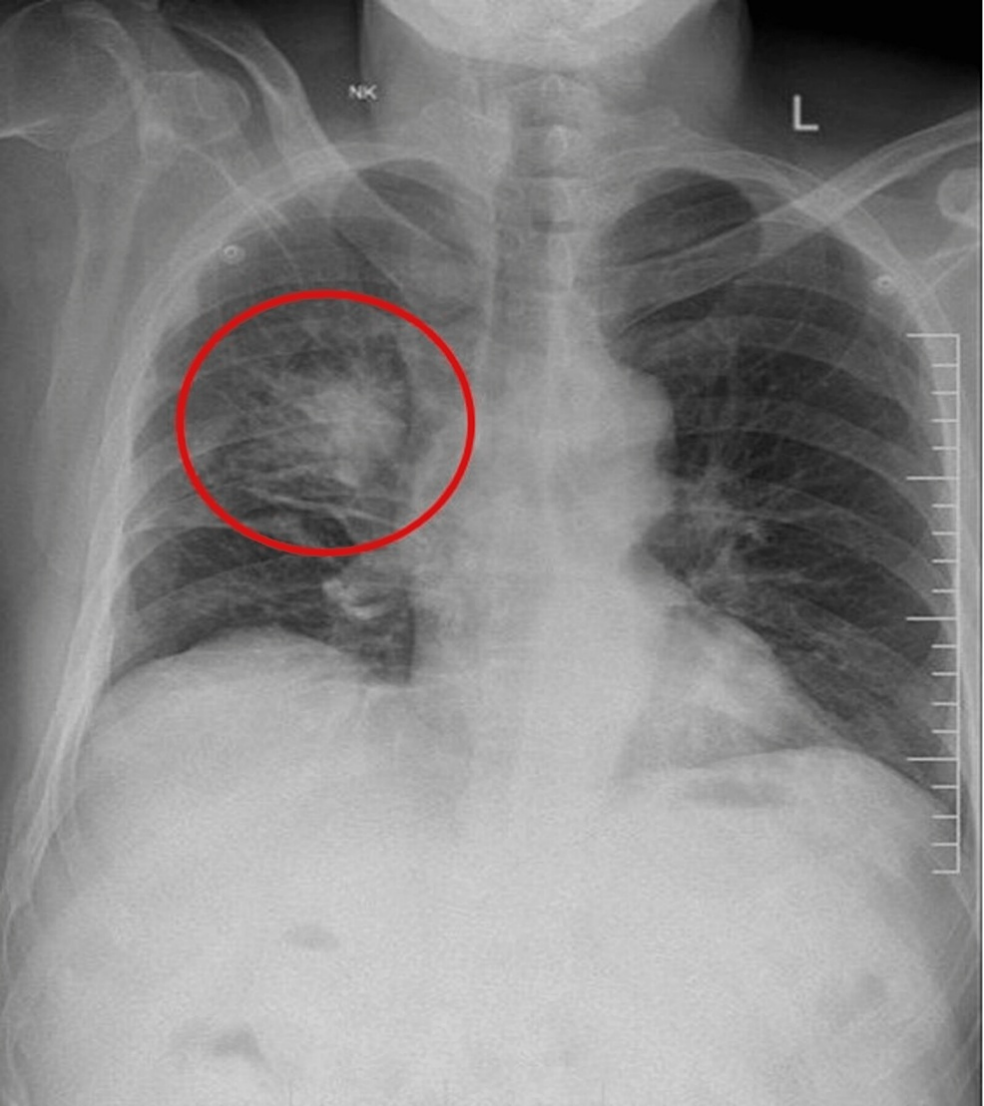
Chest X-ray PA view showing an ill-defined spiculated opacity in the right lung, malignancy (red circle) PA: posterior anterior.
